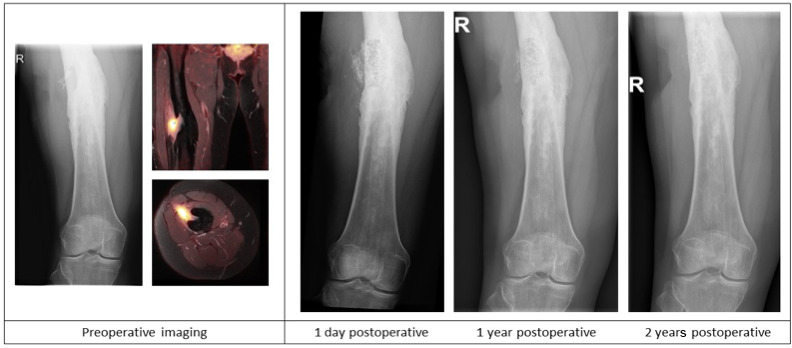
Case description of a 50-year-old female at our outpatient clinic with a painful upper leg and a draining fistula 20 years after an open femoral fracture. Preoperative X-ray and PET-MR imaging showing a chronic intramedullary osteomyelitis of the femur with a fistula and a sequester. Postoperative X-ray imaging after one-stage debridement surgery and implantation of S53P5 bioactive glass show bone defect filling
